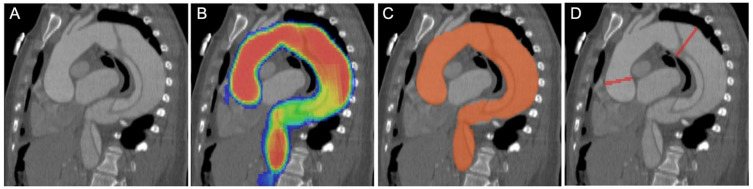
Steps involved in quantifying diameters at the widest location in each section of the aorta. (A) Candy-cane view of a thoracic aorta with a dissection in contrast-enhanced CT. (B) Probability map of the AI prediction model of the aorta. The red color shows the highest confidence for the model. Yellow, green and blue each represent a decreasing level of confidence, respectively, with red indicating the highest confidence. (C) An inclusion cutoff for the confidence level is picked, and the segmentation of the aorta can be generated based on this selection. (D) From this segmentation, the maximum diameters in each section may be calculated. AI = artificial intelligence; CT = computed tomography.
